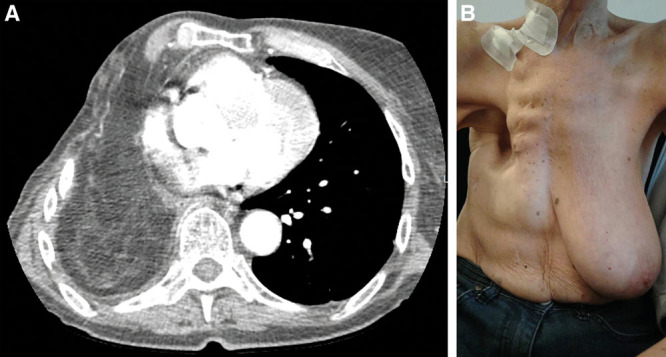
The computed tomography scan (A) shows the breast in the pleural cavity and placed against the omentum. B, The patient at 12-month follow-up.
